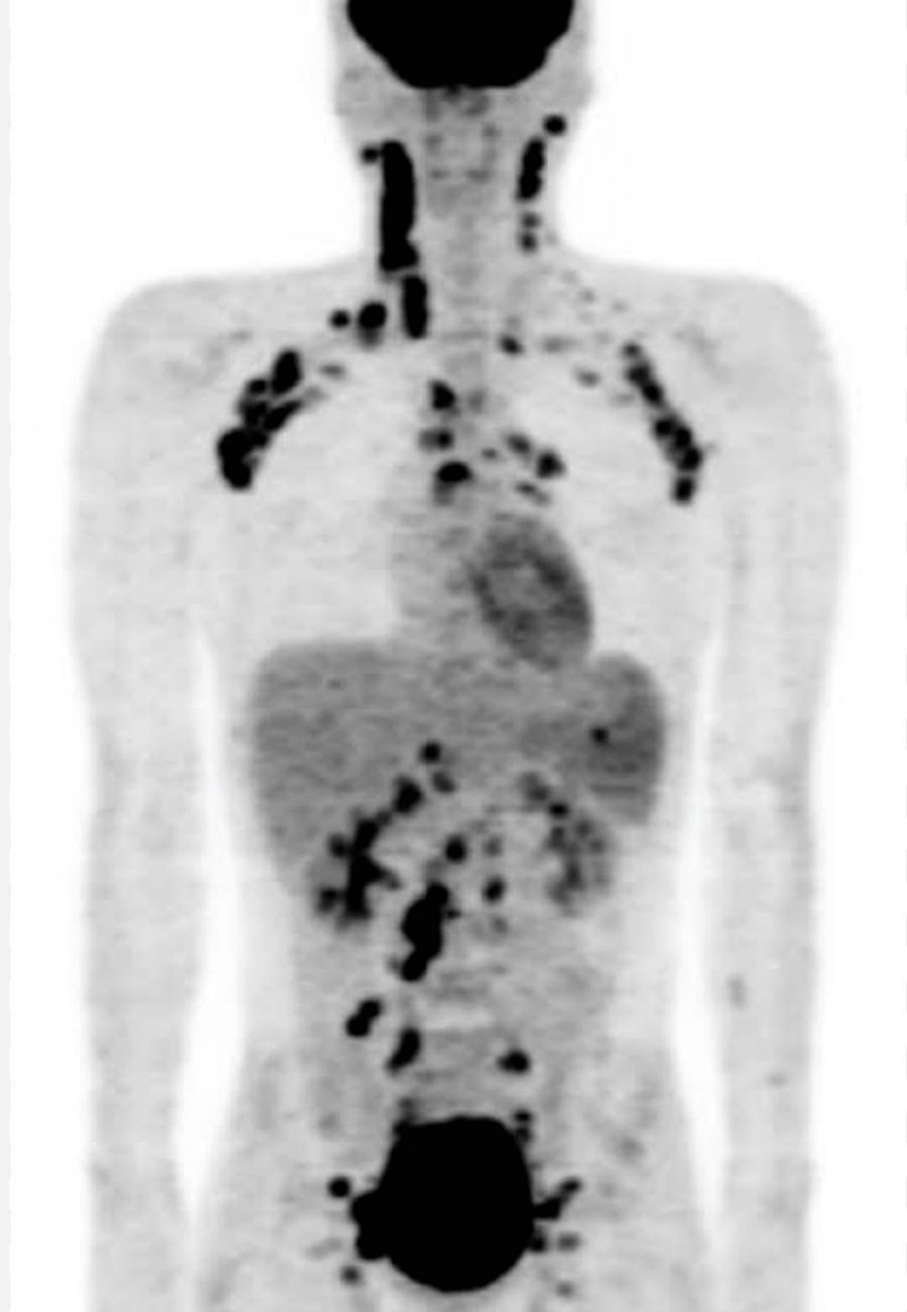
Whole-body FDG PET scan image FDG-PET: Fluorodeoxyglucose positron emission tomography
